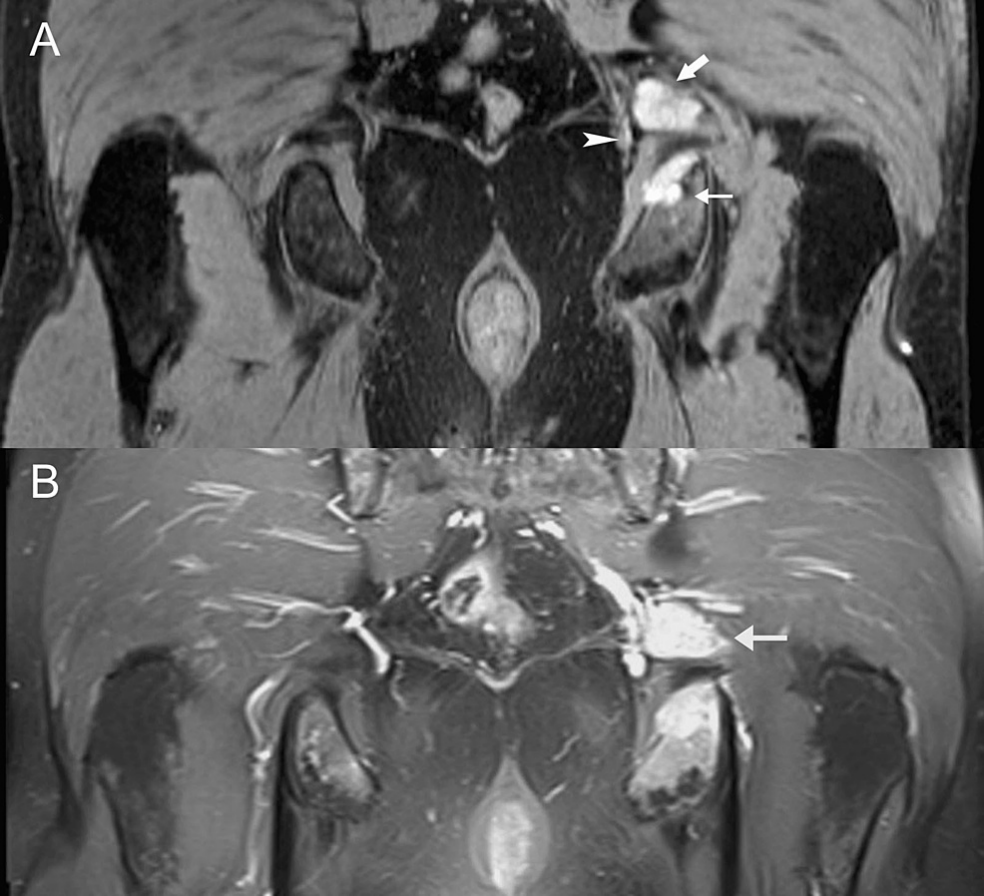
A 58-year-old man with advanced metastatic disease due to the spread of soft-tissue sarcoma. (A) Coronal high-resolution T2-weighted MR image shows infiltration of the bone (ischium) (thin arrow) and the piriformis muscle (arrow).  Note the descending sciatic nerve (arrowhead) adjacent to infiltrated muscle, responsible for sciatica. (B) Coronal T1-weighted MR image with fat saturation after the administration of gadolinium-containing contrast agent depicts ample enhancement of metastatic deposits in both the muscle (arrow) and bone. Abnormal enhancement of the sciatic nerve is also seen.
